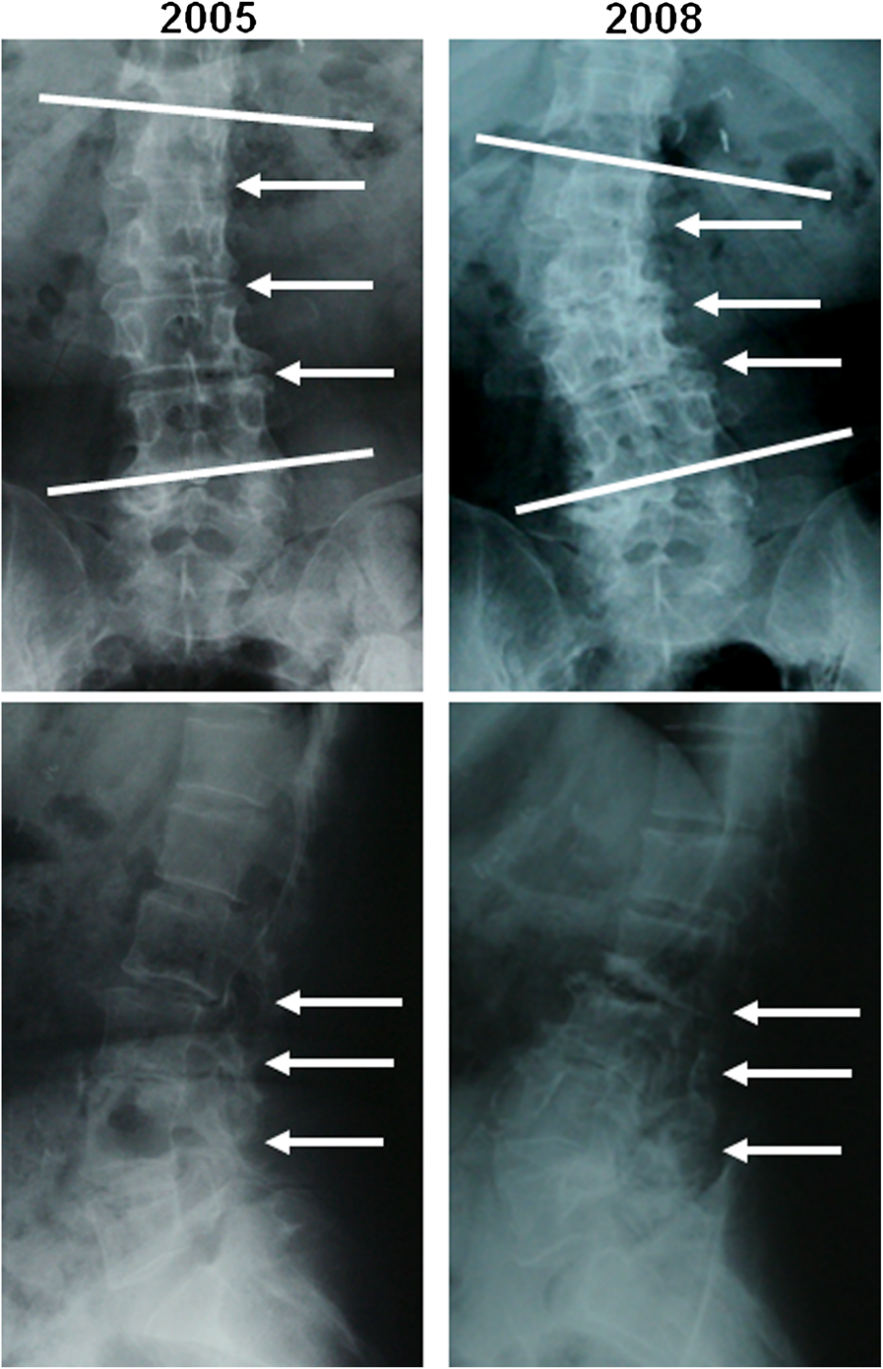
This patient is a 70-year-old woman with RA and scoliosis with a 24-year history of RA. Progression of scoliosis and changes of radiological findings were evaluated between 2005 and 2008. The radiographs taken in 2005 (*left panels*) and 2008 (*right panels*) are shown. The *lines* in the anteroposterior views (*upper panels*) indicate the upper and lower tilting vertebrae. The *arrows* of both anteroposterior and lateral (*lower panels*) views indicate progression of disc space narrowing and endplate destruction.
